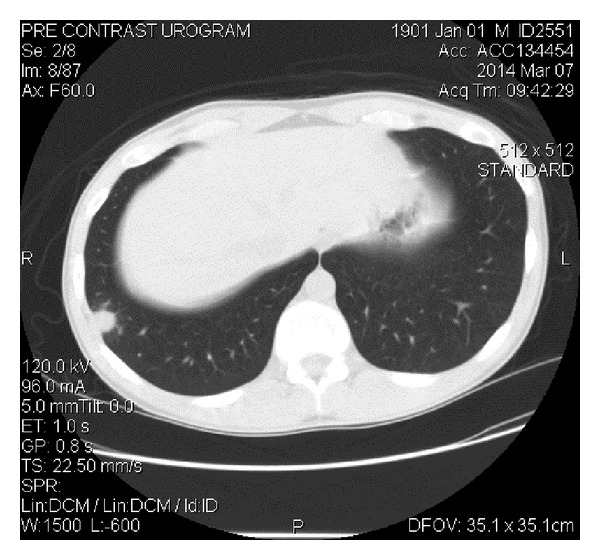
CT chest demonstrates right lung base nodule.
